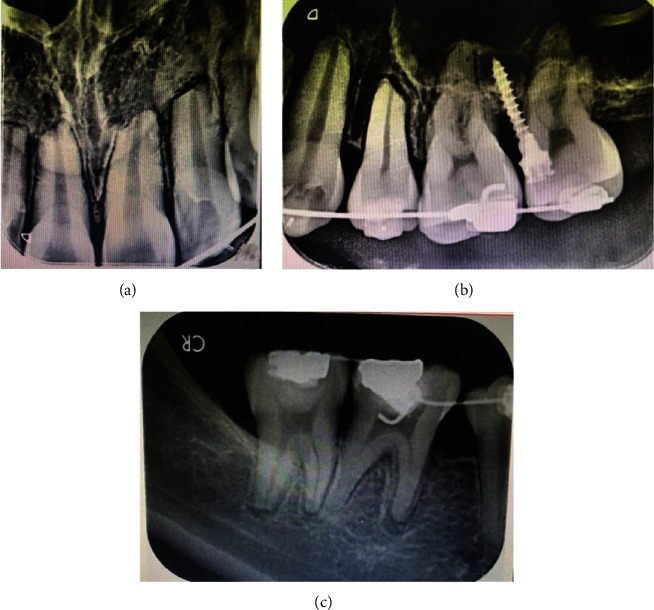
Intraoral periapical radiographs of (a) maxillary incisors, (b) maxillary left posterior teeth, and (c) mandibular right posterior teeth showing severe OIEARR.
